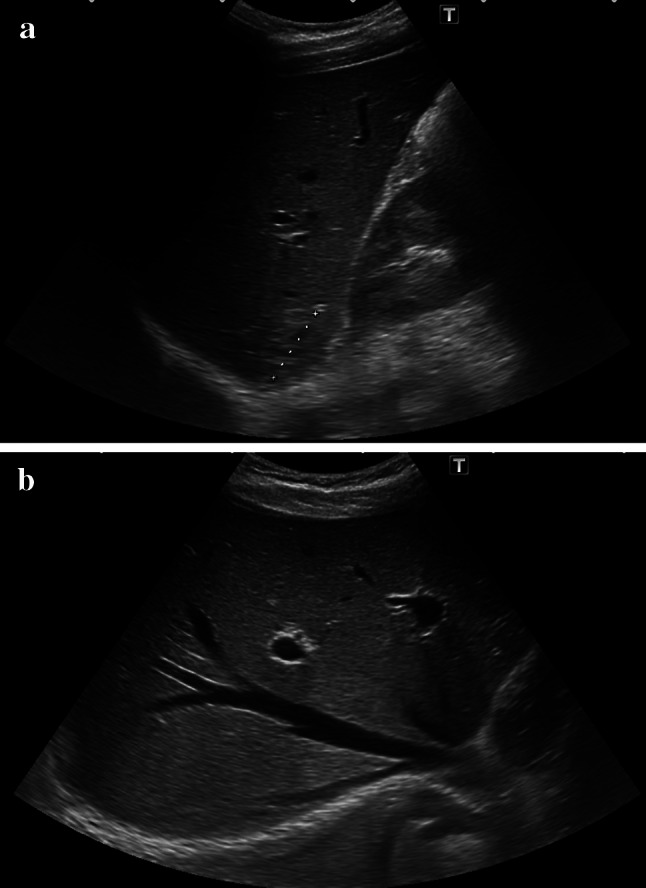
Control abdominal sonography 1 day after the HLHE showing the mass in Segment 7. a B-mode sonography showed no abnormality. b No patchy hyperechogenicity was found in B-mode
